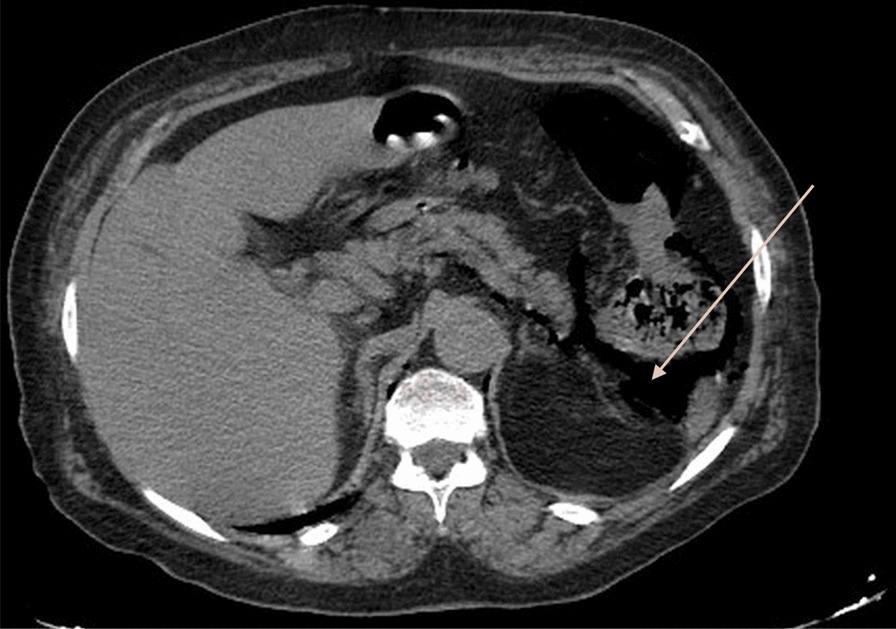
CT scan axial view showing pneumoperitoneum. Arrow points to pneumoperitoneum
